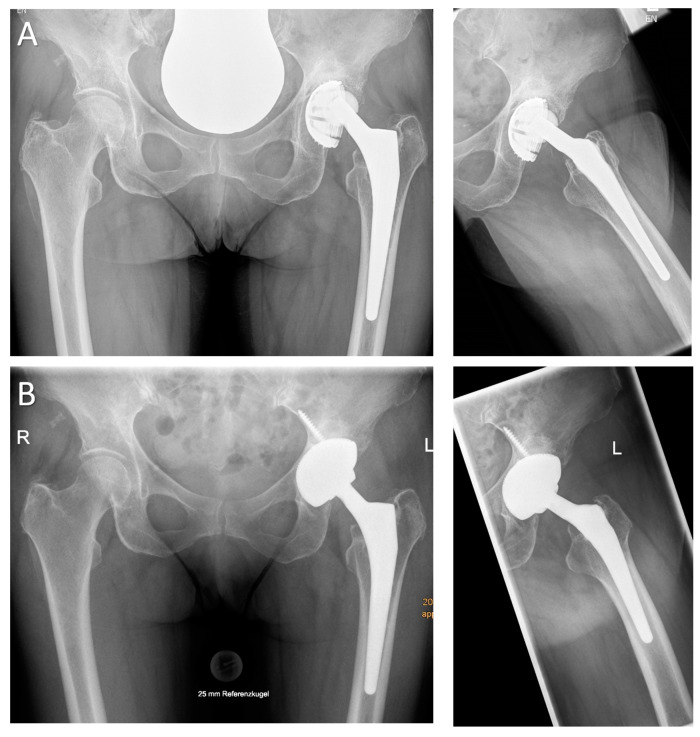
(A) A 72-year-old female patient presenting with significant polyethylene wear and aseptic loosening of the acetabular component which was implanted 11 years ago (antero-posterior and axial views). The intraoperative bone defect evaluation revealed a cranial and lateral segmental defect with supportive dome and acetabular rim (Paprosky type IIB). (B) Radiographic imaging 12 months postoperatively (a.p. and axial views) demonstrate a well-fixed asymmetric acetabular component filling the cranial defect. The distal positioning of the insert makes it possible to reconstruct the anatomical center of rotation and to treat any pre-existing leg-length discrepancy (HHS: 56 preoperatively, 91 postoperatively; operation time OT 72 min).
